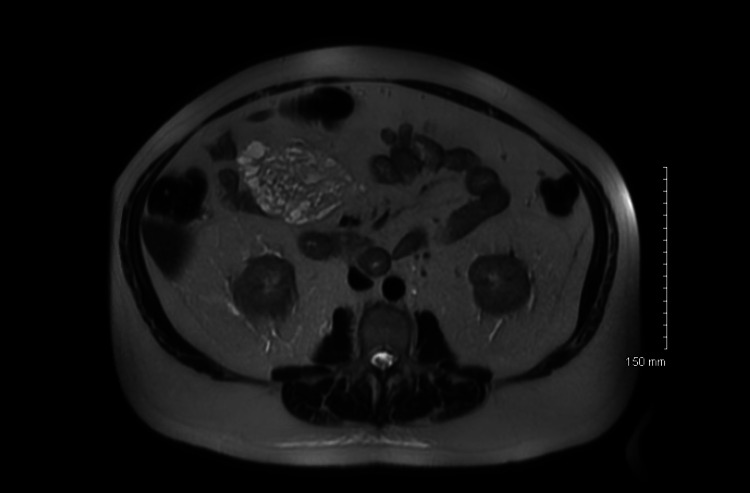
Axial image of T2-weighted MRI of right upper quadrant lymphangioma with multiple loculations.
